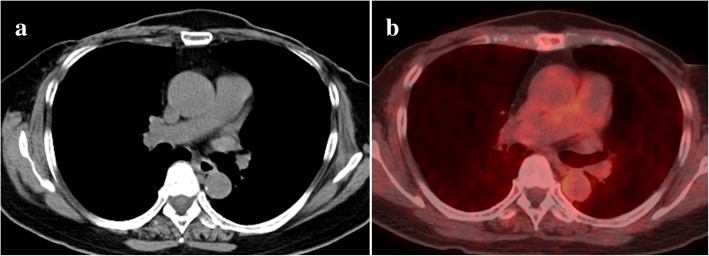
CT showing shrinking of the mass in sternum (a). The hot uptake on PET disappeared (b)
